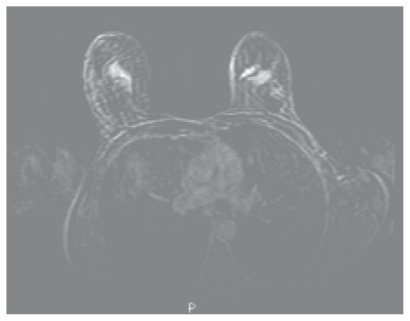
Dynamic contrast enhanced Breast MRI following 4 cycles FEC demonstrates continuing malignant enhancement in the left upper outer breast but a new enhancing lesion in the right upper outer breast, these two areas have similar malignant type contrast enhancement characteristics.
